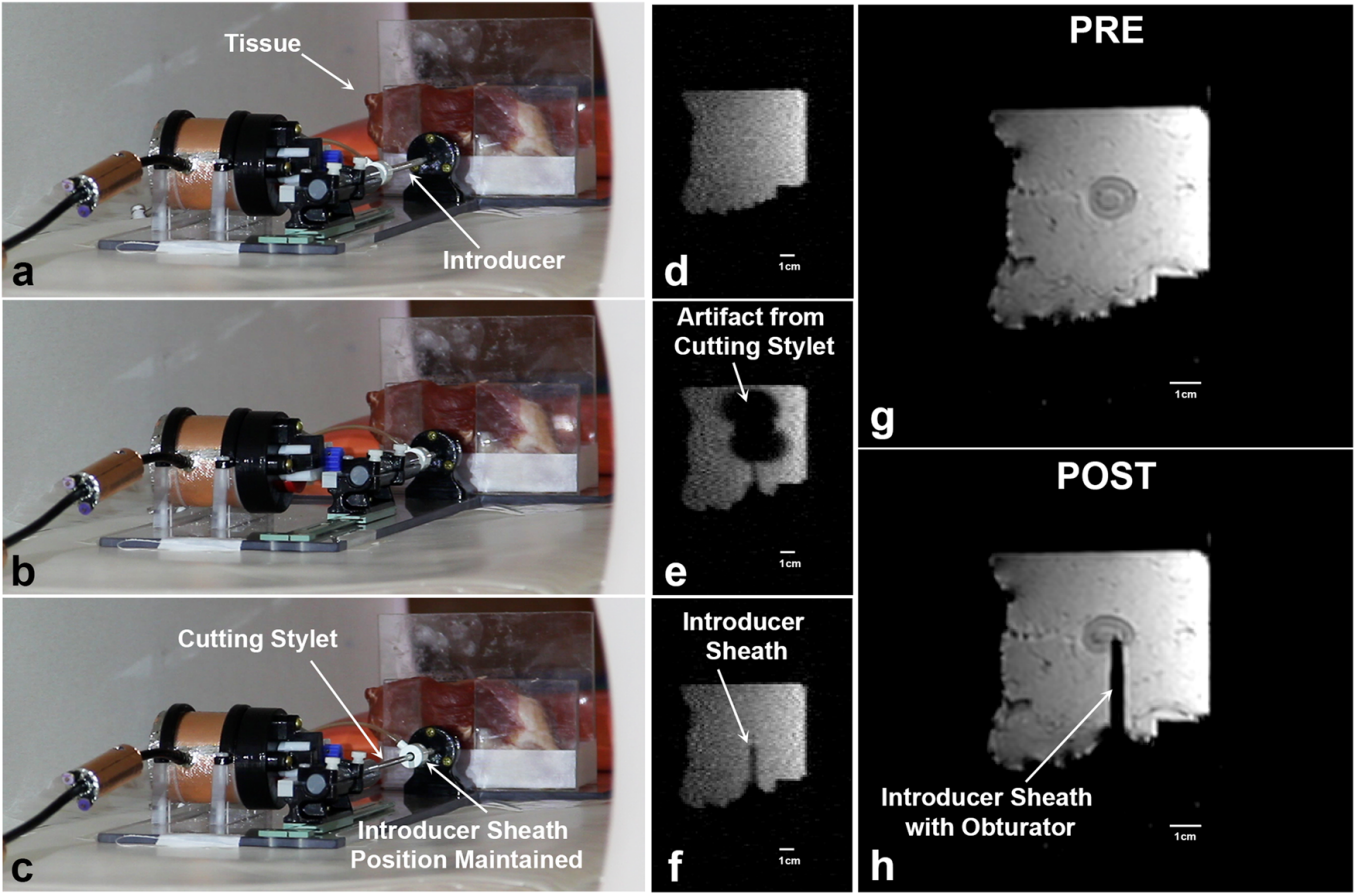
Biopsy introducer robot with MRI-compatible servomotor places introducer sheath at desired target location in ex vivo tissue. Photographs of introducer robot prior to introducer insertion (a), at target insertion depth (b), and during cutting stylet removal (c) are shown. The corresponding simultaneous MR images obtained during robot operation are shown in (d), (e), and (f), respectively. g Sagittal MR image of tissue sample showing target lesion prior to biopsy introducer placement. h Sagittal MR image following introducer sheath placement showing that desired placement position of introducer sheath was achieved.
